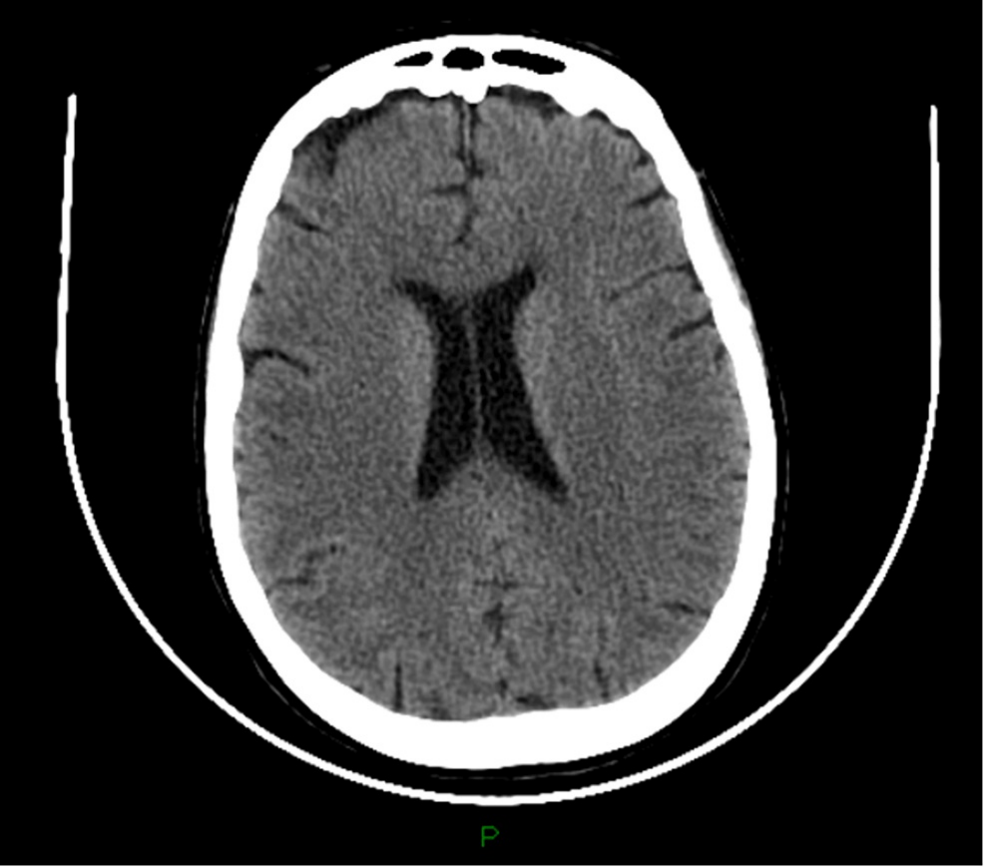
CT head No intra- or extra-axial hemorrhage. No evidence of acute large vessel infarct. No intracranial mass effect. There is preservation of the gray/white matter interface.
